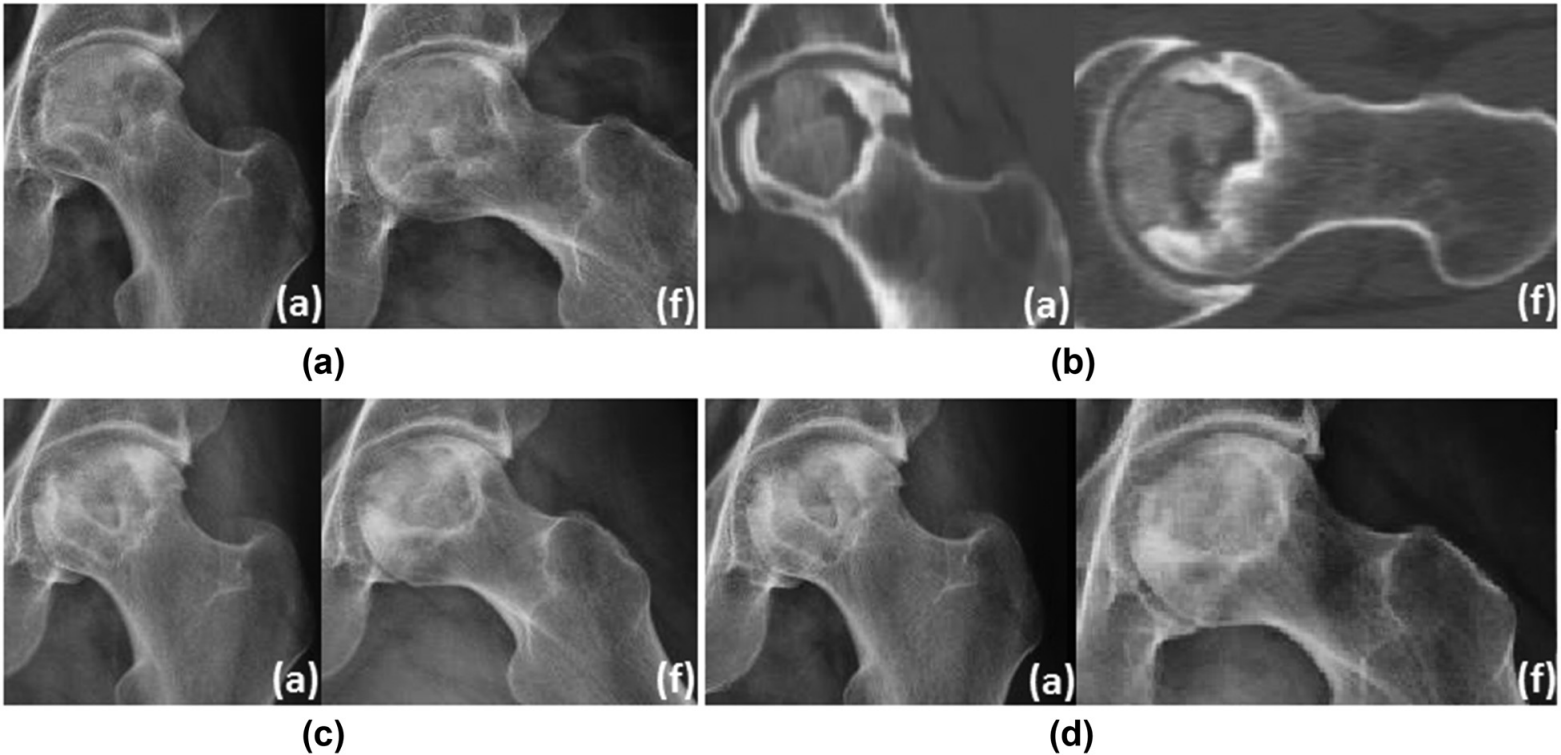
Typical case of ONFH with cystic area: (a) (radiograph imaging at an anteroposterior (a) and frog (f) lateral view showed large-scale necrosis of the left femoral head), (b) CT showed obvious cystic area, (c) 1 year follow-up, and (d) 2 years follow-up.
